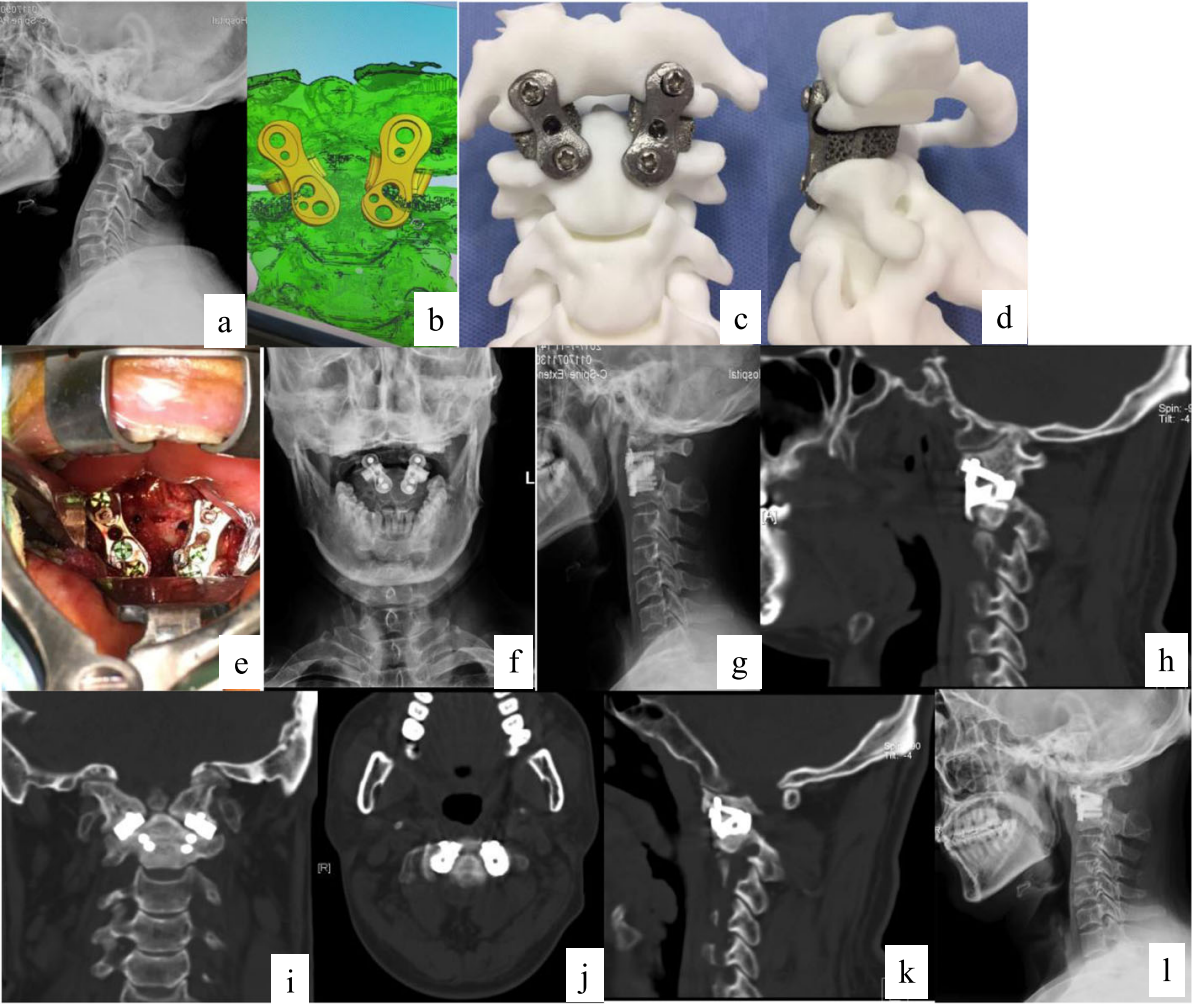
a Pre-operatively lateral X-ray; b Anterior view of the computer-aided design model of 3DPLC; c Anterior view of the manufactured 3DPLC based on 1b on a 3D-printed model; d Lateral view of the manufactured 3DPLC based on 1b on a 3D-printed model; e Intra-operative view of the 2 3DPLC before closure; f Anteroposterior X-ray 1 week after the surgery; g Lateral X-ray 1 week after the surgery; h Left sagittal reconstruction of CT of the cervical spine 12 months post-operatively; i Coronal reconstruction of CT of the cervical spine 12 months post-operatively; j Axial CT of the cervical spine 12 months post-operatively; k Right sagittal reconstruction of CT of the cervical spine 12 months post-operatively; l Lateral X-ray 18 months after the surgery
